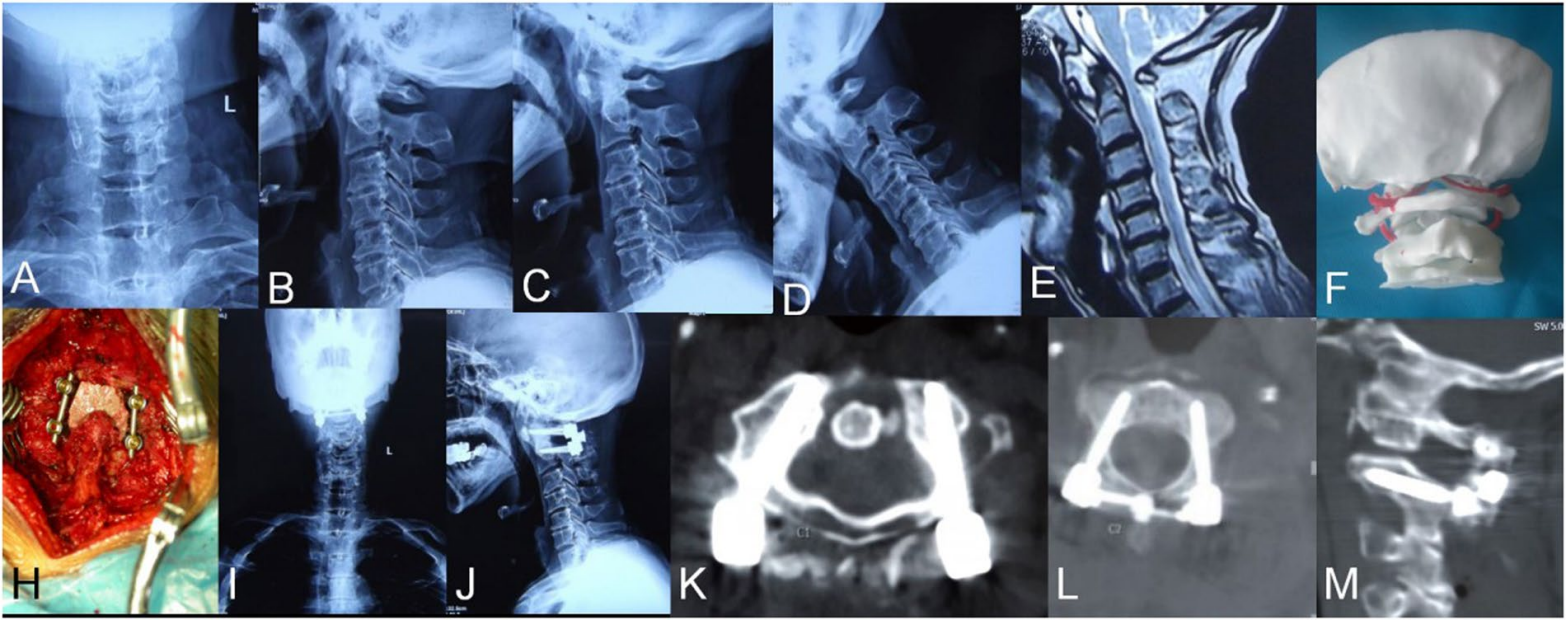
A 47 year-old male with atlantoaxial dislocation received posterior reduction and C1 pedicle-C2 pedicle internal fixation assisted by a 3D-printed life-size model. (A–D) Preoperative X-ray photographs showing atlantoaxial dislocation. (E) Preoperative MRI showing compression of the spinal cord. (F) A life-size C0-2 model. (H) Intraoperative photograph. (I–M) Postoperative photographs showing that the atlantoaxial dislocation was reduced and that the screw position was satisfactory.
